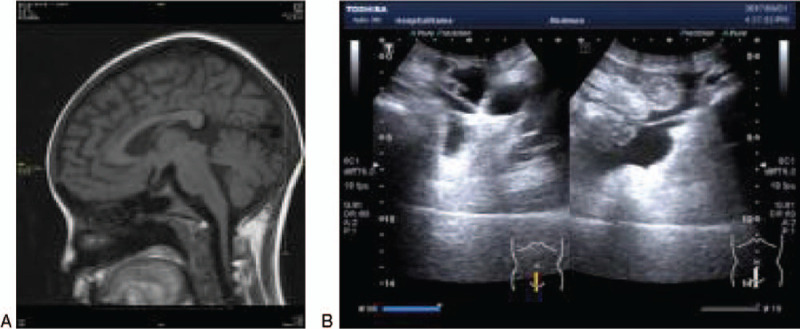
(A) Pituitary MRI revealed partial empty sella. (B) The uterus and both ovaries could not be identified by pelvis ultrasonography. MRI = magnetic resonance imaging.
